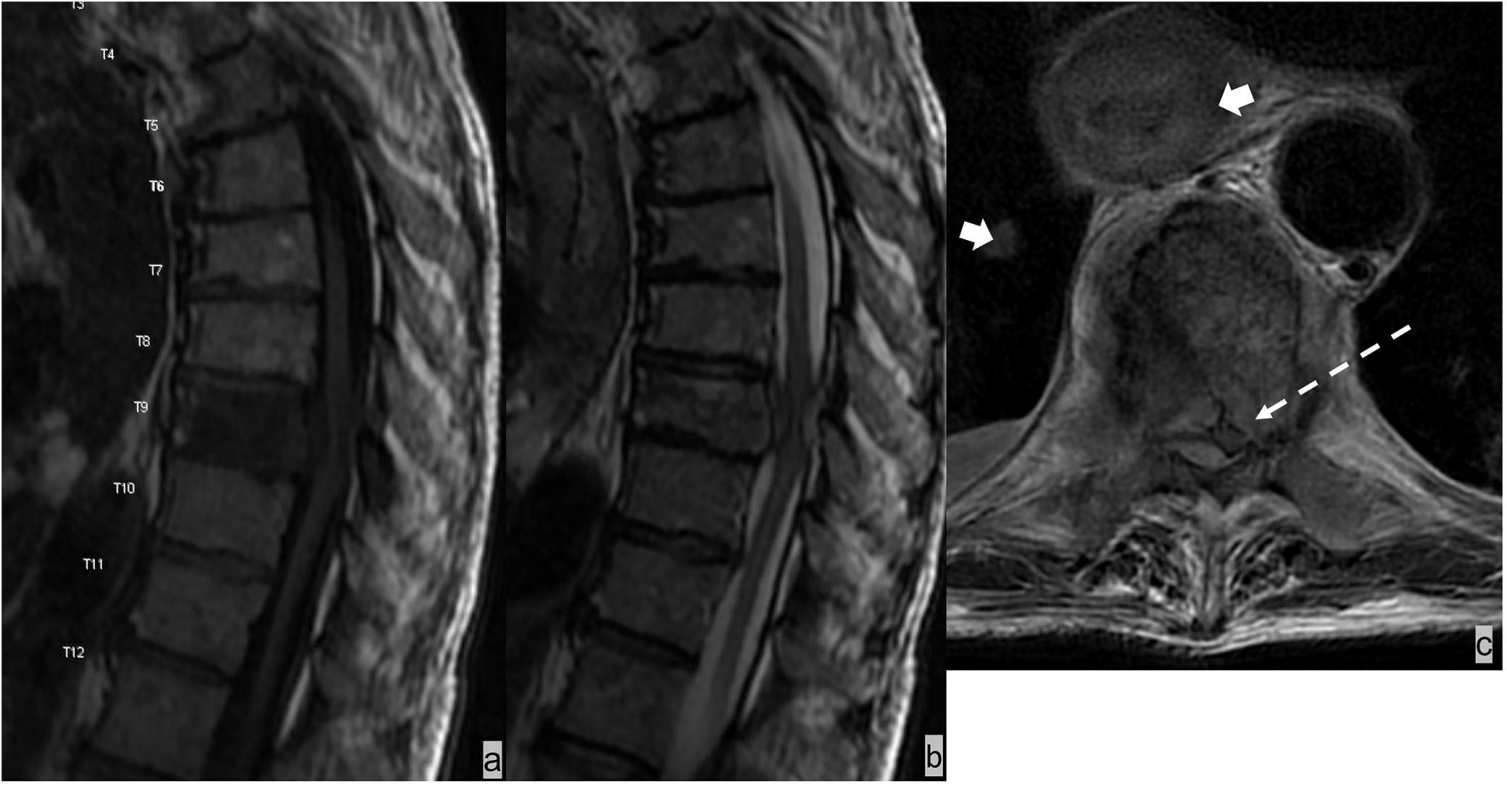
The patient had esophageal cancer with spinal metastases. Sagittal T1w and T2w sequences of the MRI lumbar spine (a,b) demonstrated marrow-replacing bony metastases with ventral and dorsal epidural extension (dotted white arrow), complete effacement of the thecal sac, and spinal cord compression at T9. This is a grade 3 epidural cord compression on the ESCC scale (c). MRI also showed mural thickening of the esophagus and lung metastases (broad white arrow).
